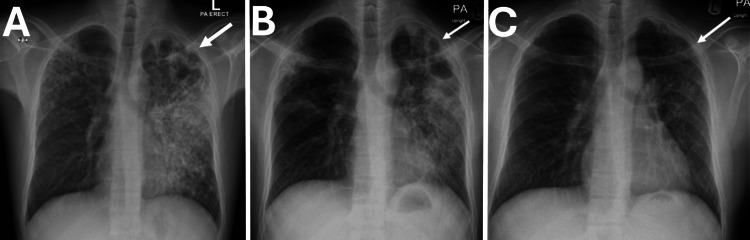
Chest X-ray showing TB treatment progression. (A) before anti-TB treatment; bilateral diffuse patchy, and nodular infiltrates more in the left lung with apical cavitations. Tuberculosis should be considered. (B) After discharge; regression of infiltrates two-months after anti-TB treatment. (C) Six‑month follow‑up; near complete resolution of lesions
